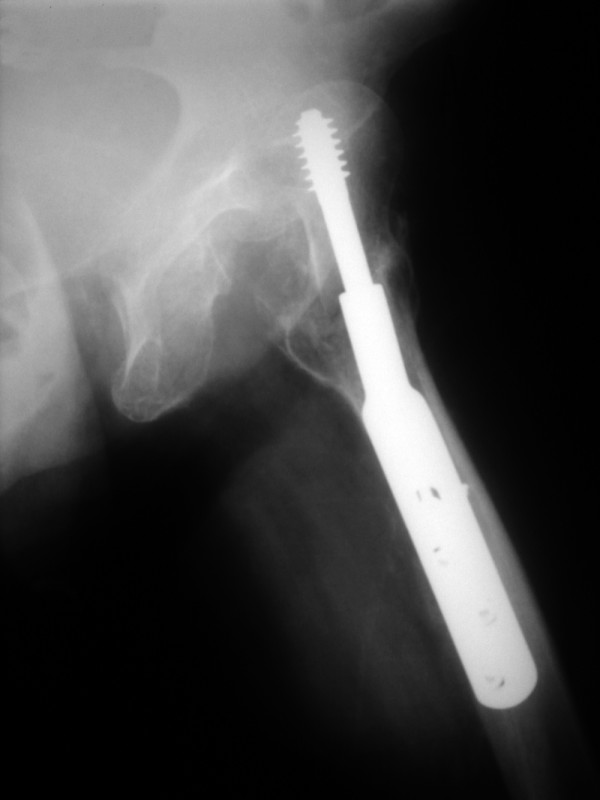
X-ray of the left hip demonstrating a posterior hip dislocation.
